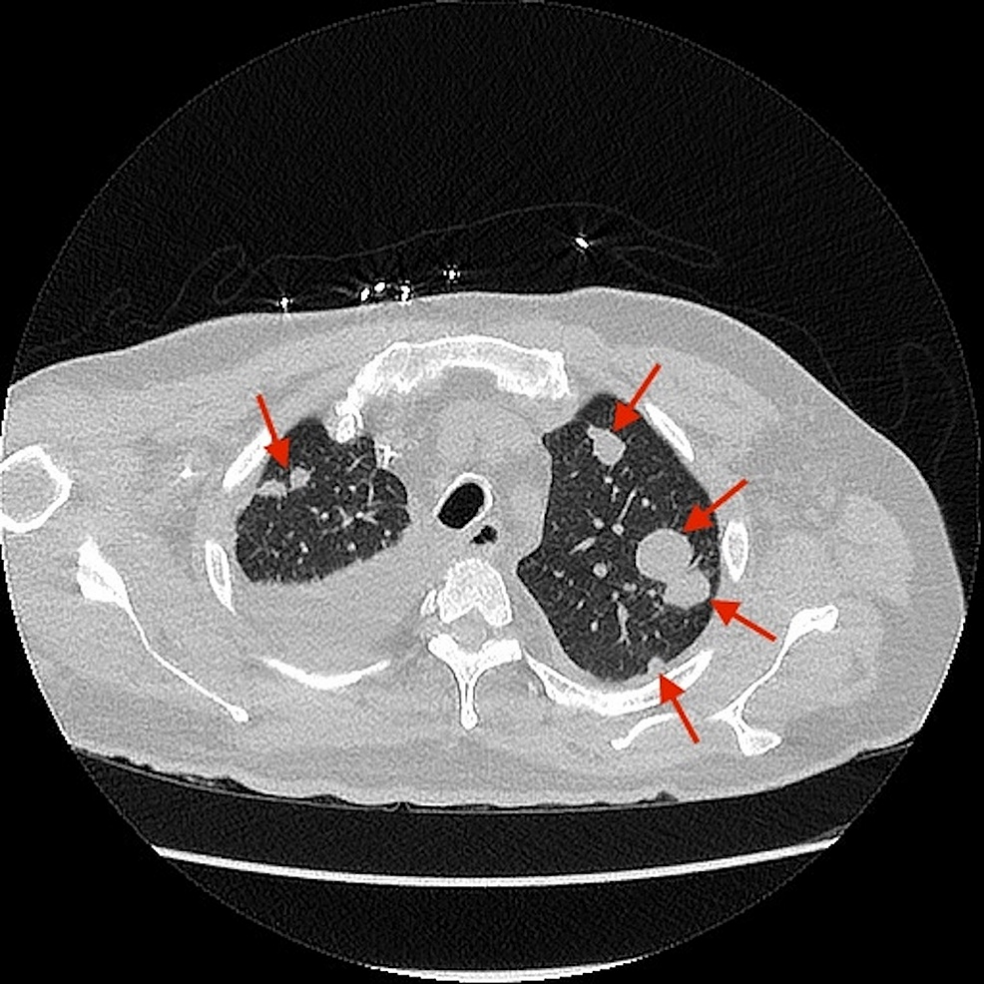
CT scan of the chest with contrast showing multifocal infiltrates throughout the lungs (arrows) CT: computed tomography
